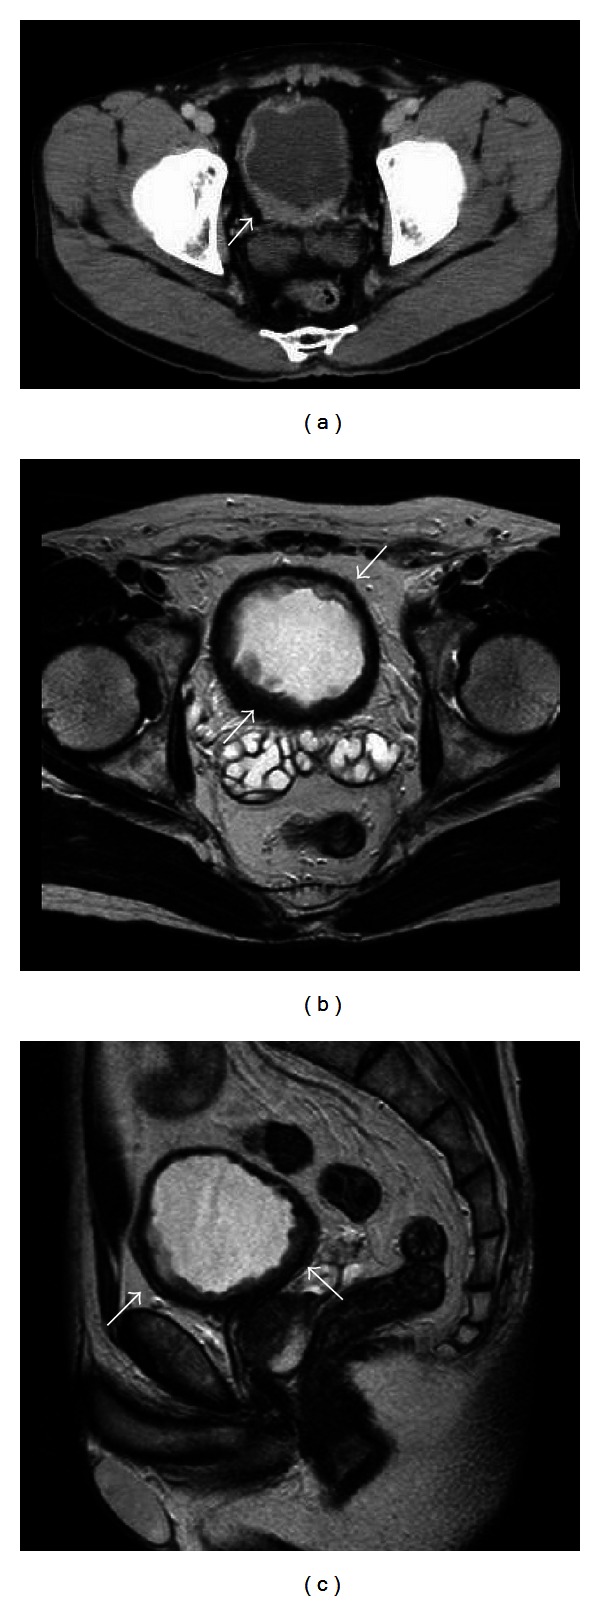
(a) CT shows diffuse tumor of the urinary bladder wall. (b)Transverse section of MRI demonstrates near-circumferential urinary bladder wall thickening. (c) Sagittal section of MRI shows urinary bladder wall thickening from anterior to posterior wall.
